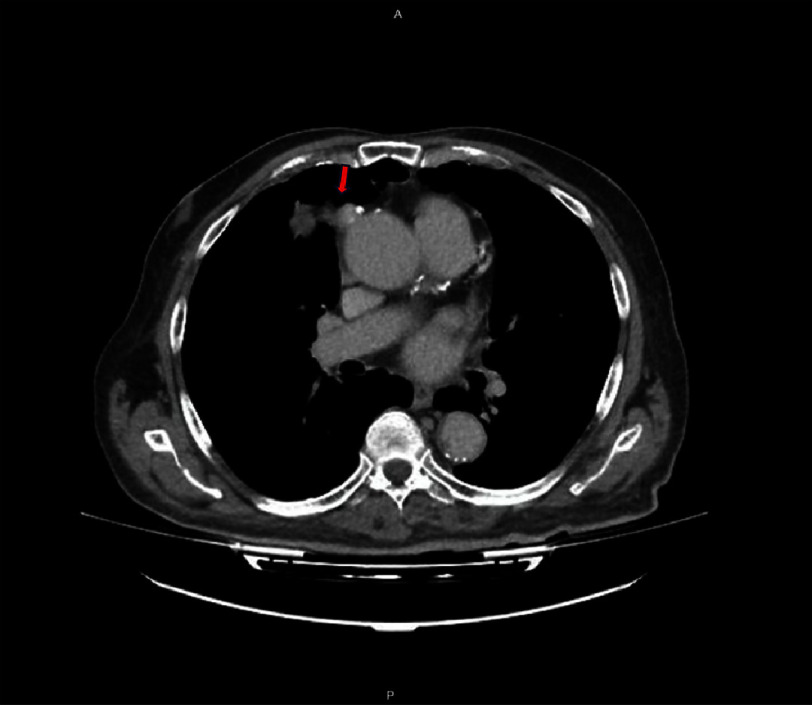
Computed tomography angiography of the thorax with intravenous contrast, demonstrating rupture of the saphenous vein graft proximally and formation of a pseudoaneurysm-red arrow.
